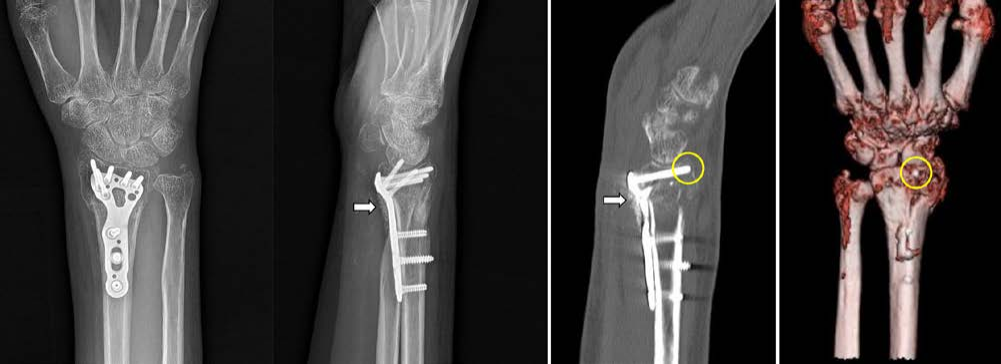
Plain radiographs and computed tomography demonstrated that one of the distal screws of the volar plate was protruding beyond the dorsal cortex (yellow circle), and radiopaque material was visible along the volar-radial aspect of the plate (white arrow).
